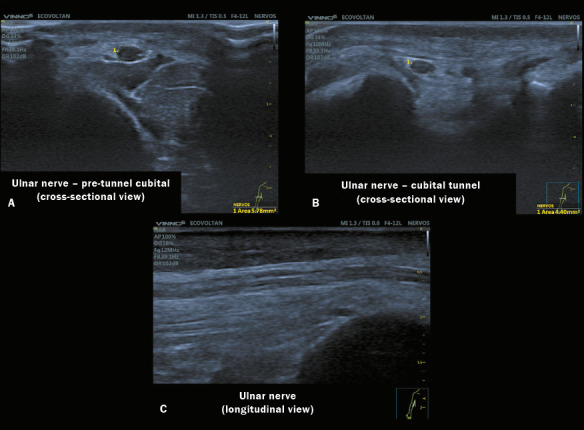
High-resolution neuromuscular ultrasound images of three sites of the same ulnar
